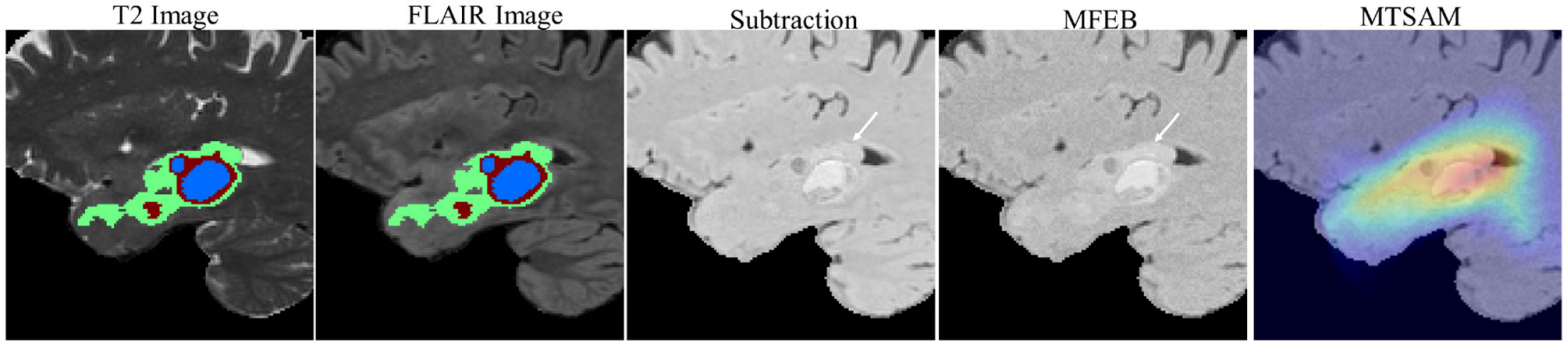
Visualization of T2-FLAIR mismatch feature.
